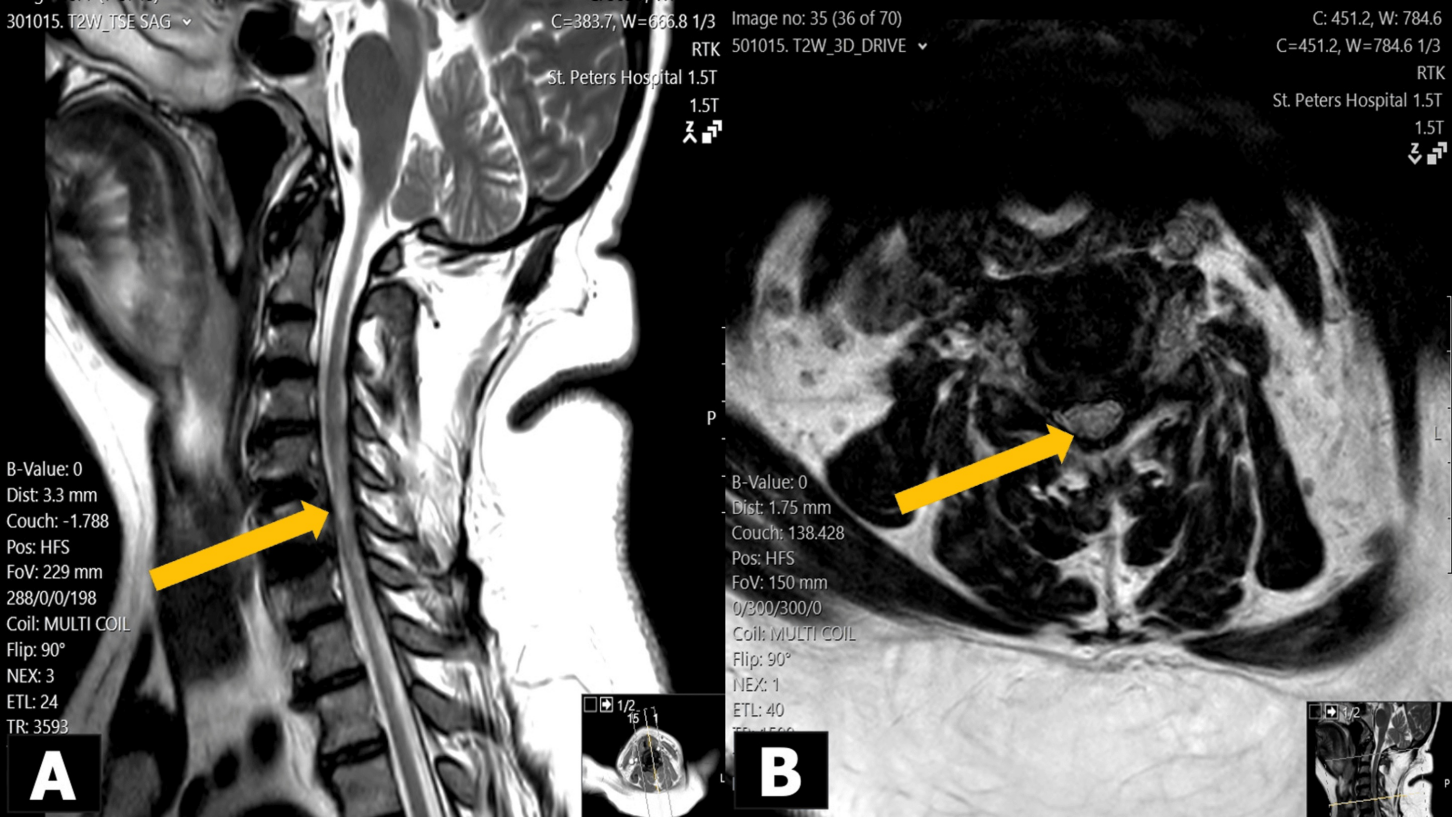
Postoperative T2-weighted MRI scans after six weeks showing persisting myelomalacia most prominent at C5/C6 level (yellow arrows) but with no acute cord compression in a) sagittal view and b) axial view.
